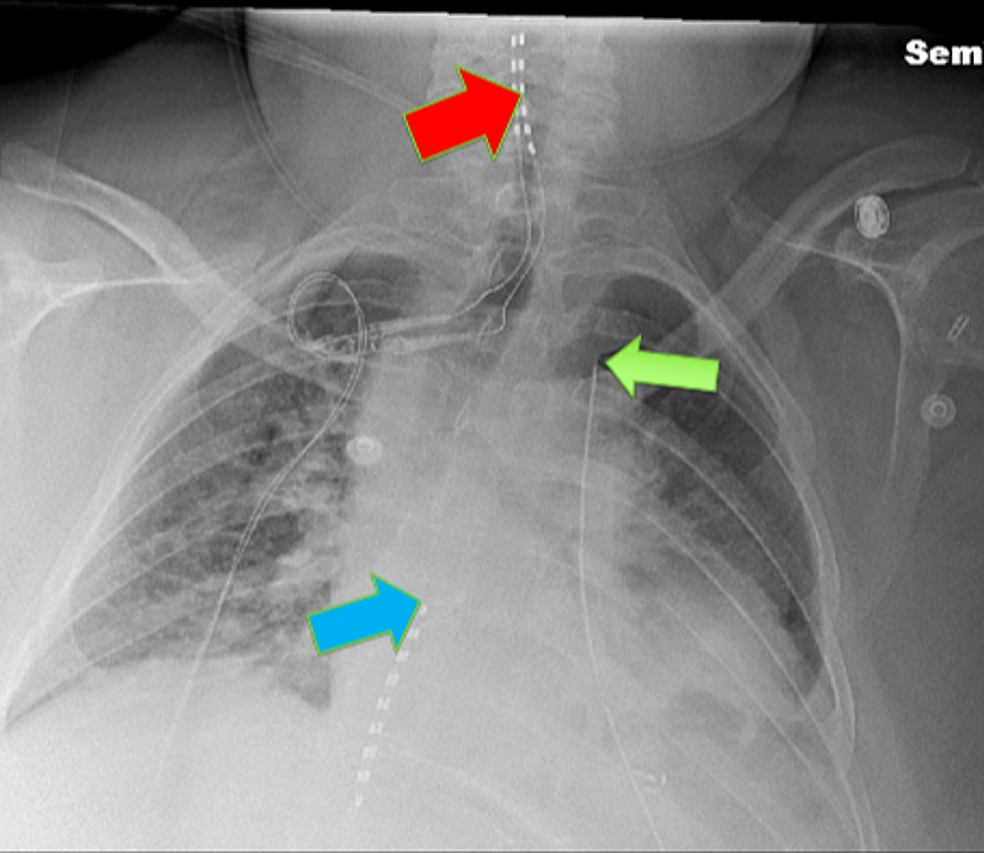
Postoperative chest radiograph A postoperative chest radiograph was obtained on postoperative day one. The cervical spinal cord stimulator has two cylindrical leads (red arrow) which can be visualized extending to the C2 vertebral body proximally and tunneled inferiorly to a presumed right-sided generator (not visible). The thoracic spinal cord stimulator has two cylindrical leads (blue arrow) which can be visualized at the superior endplate of the T7 vertebral body with its distal lead tunneled inferiorly towards to left-sided generator (not fully visible). The erector spinae plane catheter (light green arrow) overlies the left T3 to T6 transverse processes. The catheter is directed cranially with its tip terminating between the T3 and T4 transverse processes.
